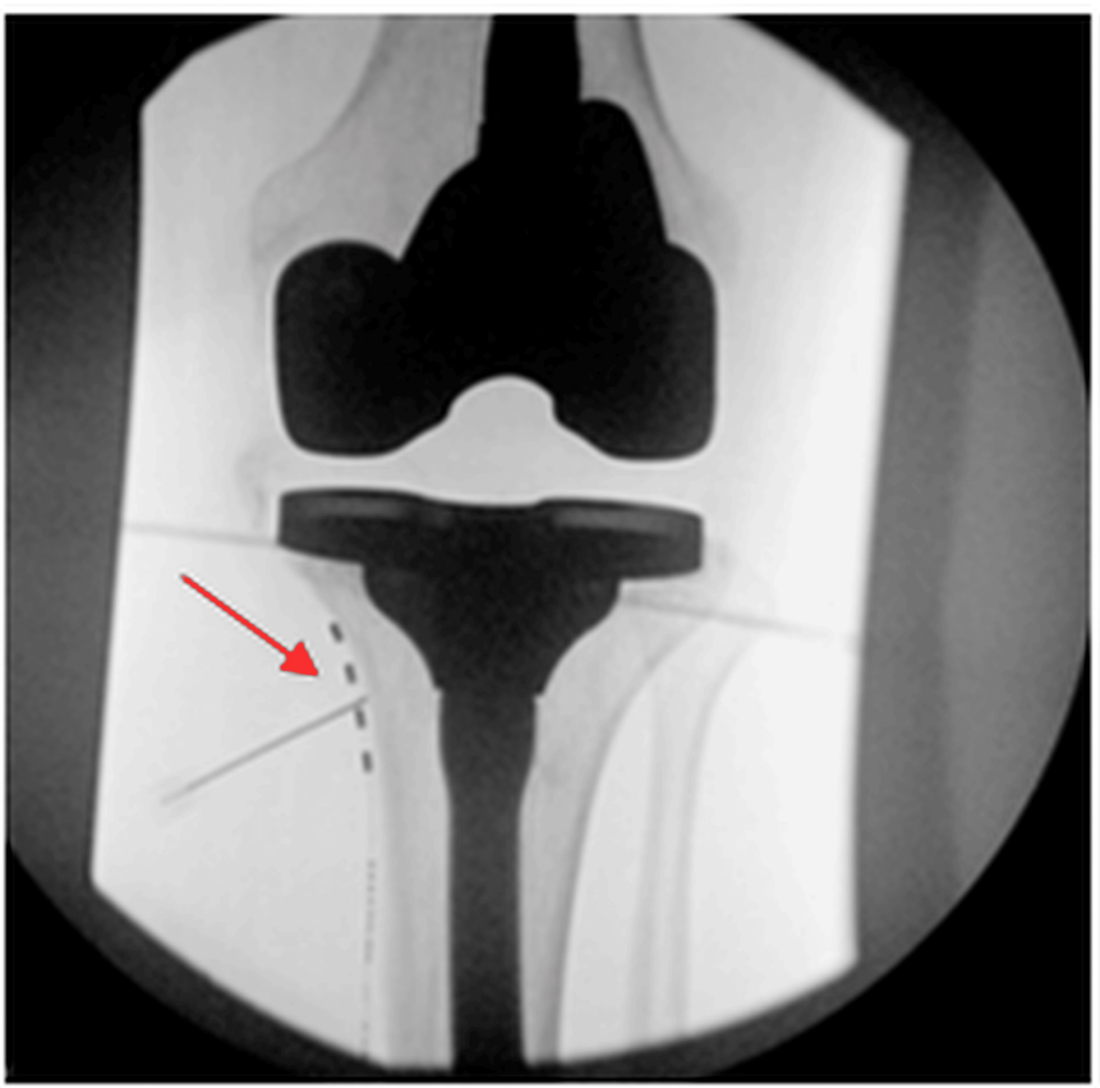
Anteroposterior radiograph of the permanent placement of the peripheral nerve stimulator (PNS) targeting the left inferomedial genicular nerve. The lead (indicated by the red arrow) is positioned along the tibial midshaft and flare.
